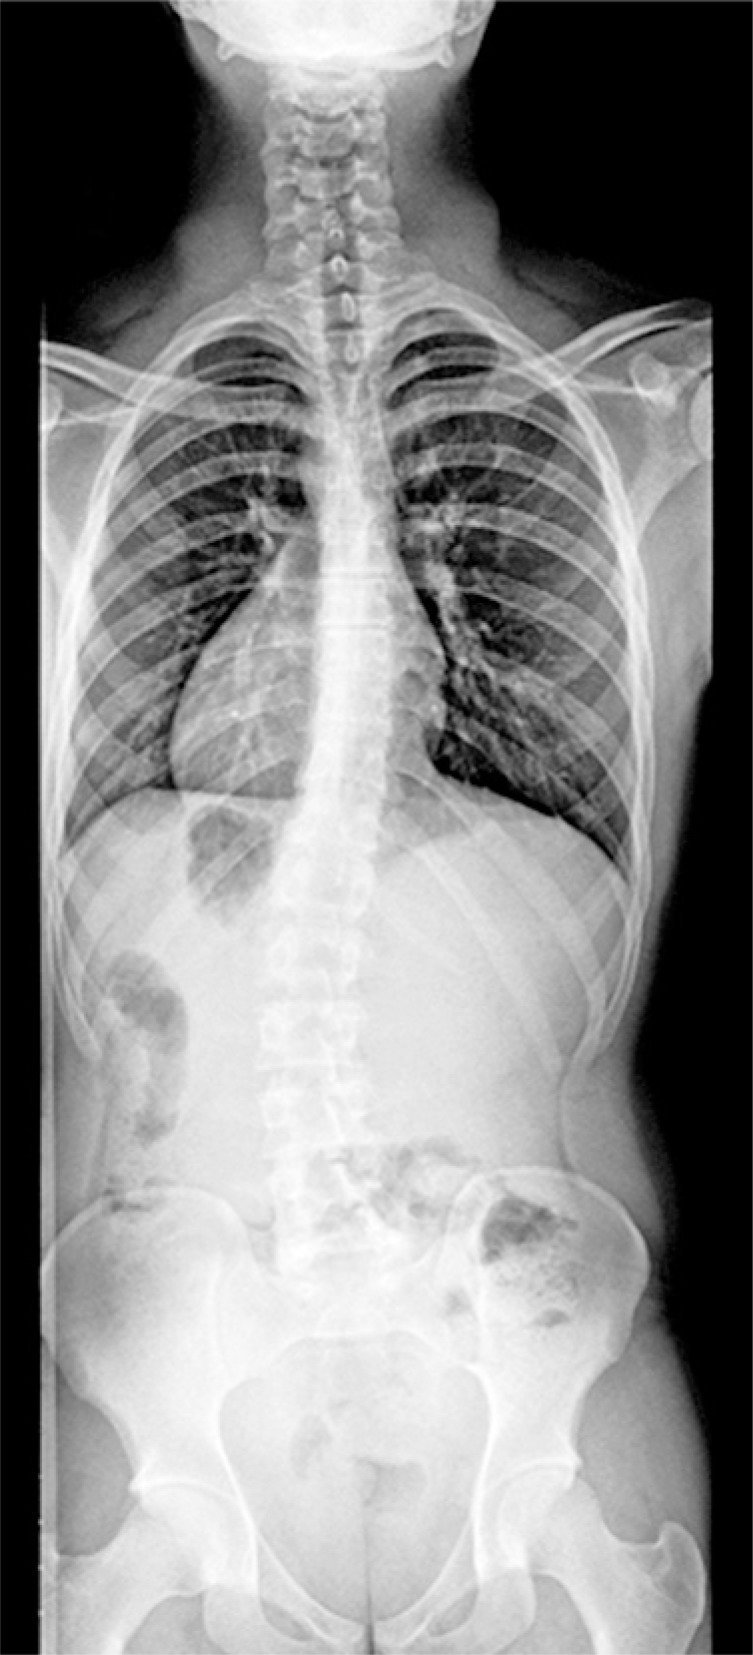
Panoramic radiograph of the total spine showing thoracolumbar sigmoid scoliosis and dextrocardia
